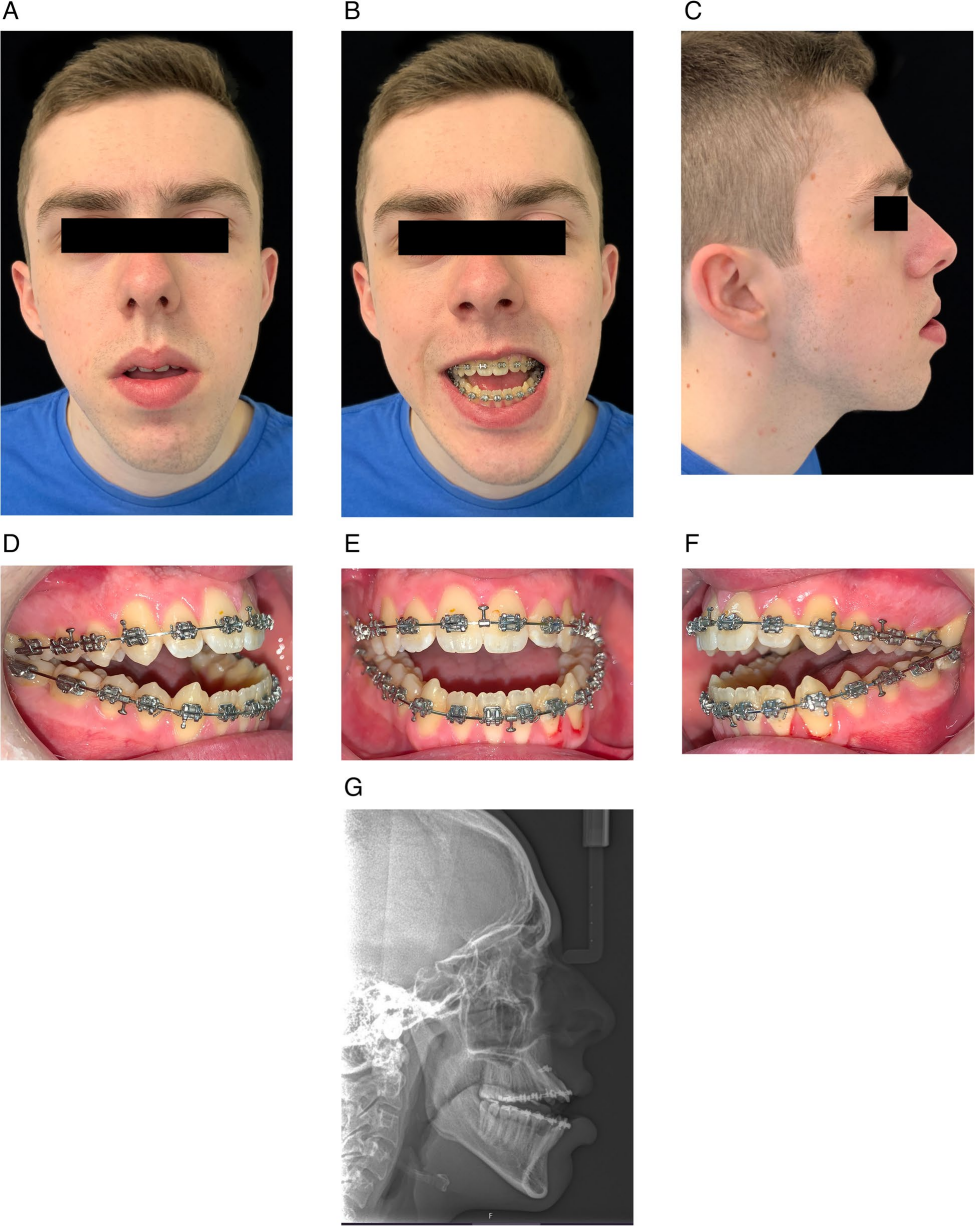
Preoperative clinical photographs and lateral cephalometric radiograph
